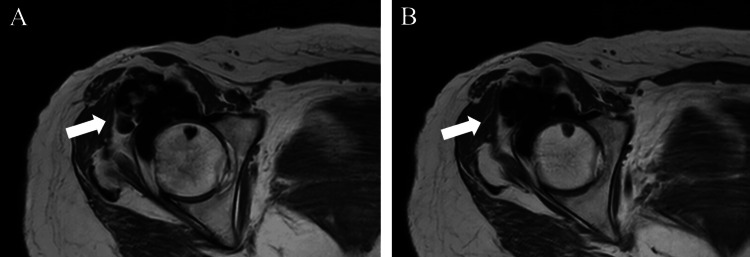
T1-weighted (A) and T2-weighted (B) images of the mass (white arrow) on magnetic resonance imaging (MRI) of the right hip.
